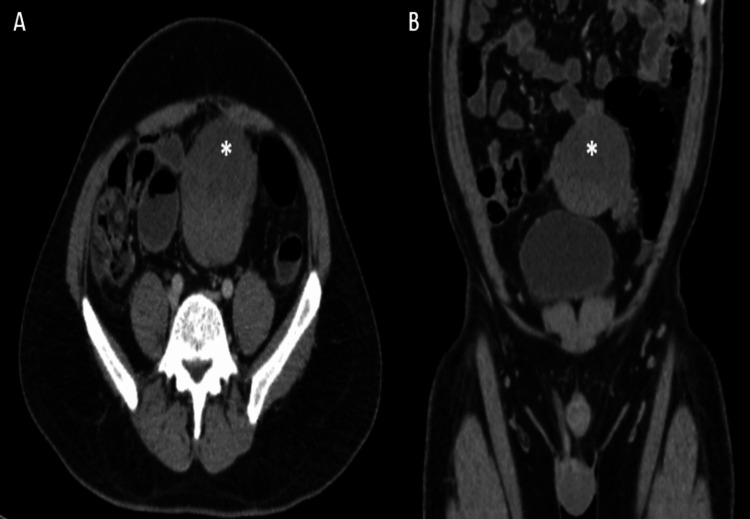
Axial (A) and coronal (B) sections of post-contrast CT show heterogeneous post-contrast enhancement of mass and non-enhancing necrotic components (asterisk)
